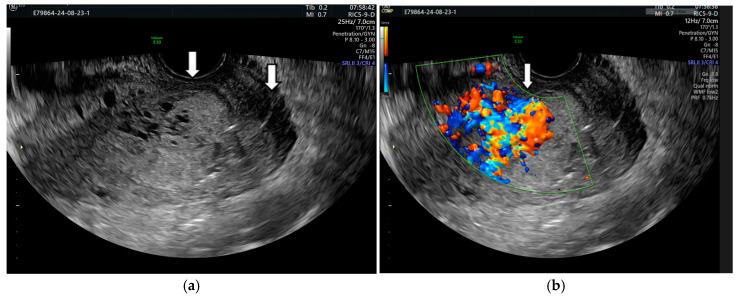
Vaginal ultrasound image of the uterus. Arrows show (a) multiple anechoic areas in the myometrium and uterine cavity with hematometry; (b) marked vascularity with a colored mosaic pattern.
